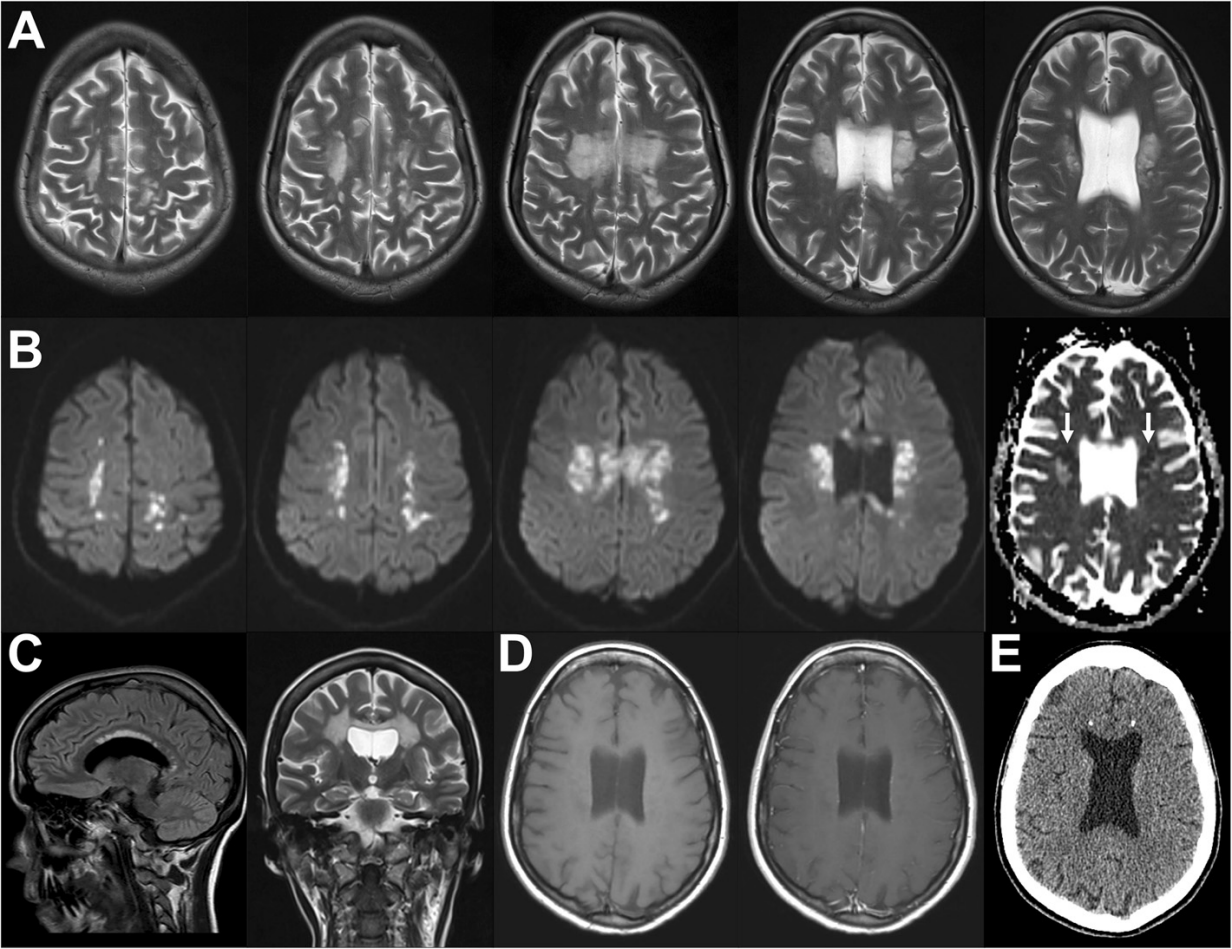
Neuroradiological findings. a MRI shows confluent hyperintense changes of the white matter on T2-weighted images, predominantly involving the pyramidal tract. b All areas displayed restricted diffusion, last image in row shows decreased values on ADC (white arrows). c Involvement of the corpus callosum on sagittal FLAIR (left) and coronal T2-weighted images (right). d Lack of contrast enhancement on T1-weighted images before (left) and after application of gadolinium (right). e On computed tomography two spots of calcification are seen in both frontal lobes
